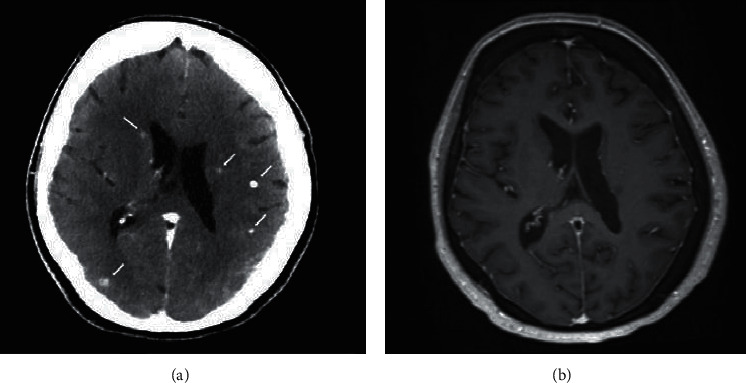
Dynamics of brain metastases on lorlatinib. Axial brain CT showing brain metastases (white arrows) after lorlatinib dose reduction (a). Axial brain MRI (T1 with contrast) performed two months after resuming full dose lorlatinib showing complete resolution of all brain lesions (b).
